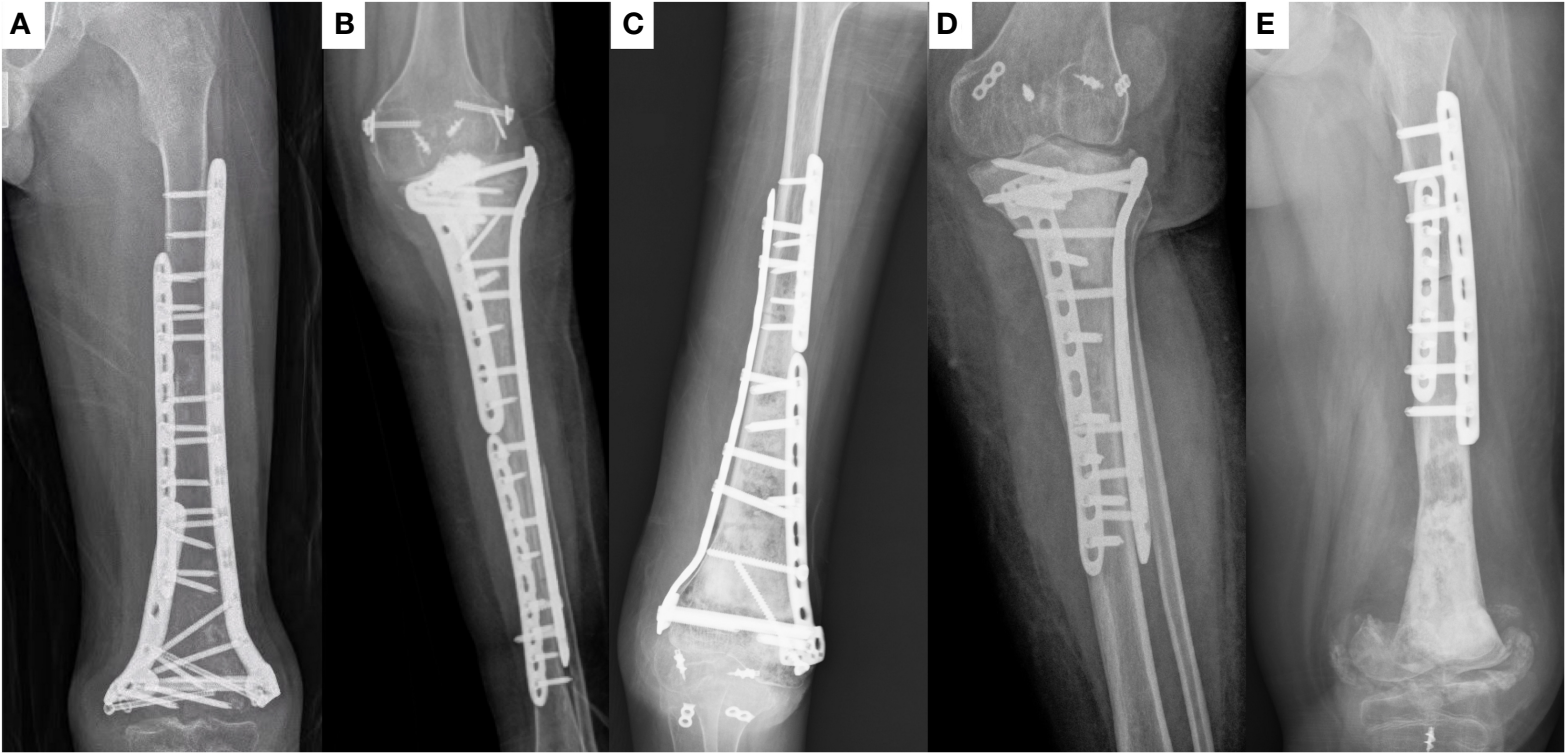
X-ray of patients with joint deformity. All five patients (case 6, 9, 17, 19, and 38, respectively) with inactivated bone containing the knee joint surface experienced this complication.
